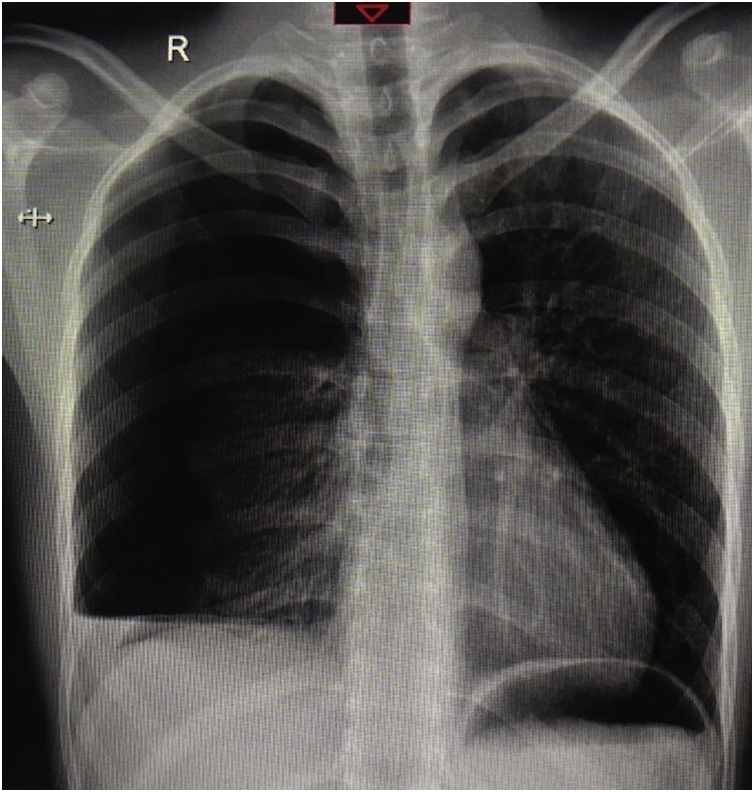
Initial chest x-ray posterior anterior view showed right apical pneumothorax with air fluid level and a collapsed lung.
